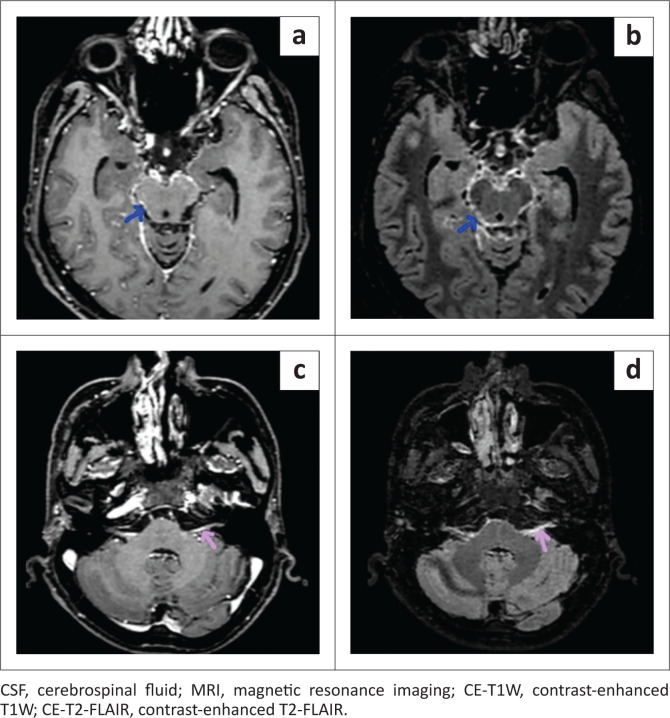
Axial brain MRI of a 30-year-old male patient with CSF proven meningitis. Basal cisternal enhancement (blue arrow) and cranial nerve enhancement (pink arrow) are more prominently seen on CE-T2-FLAIR (b and d) than on CE-T1W (a and c).
